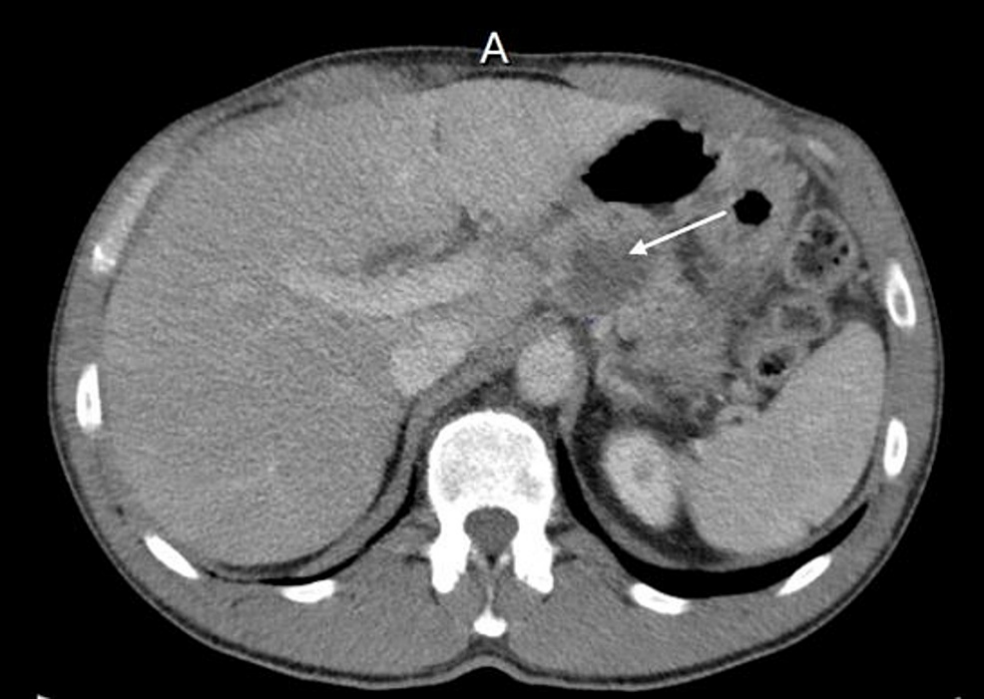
CT abdomen revealing a lesion (arrow) in the pancreas concerning pancreatic malignancy versus a pseudocyst.
